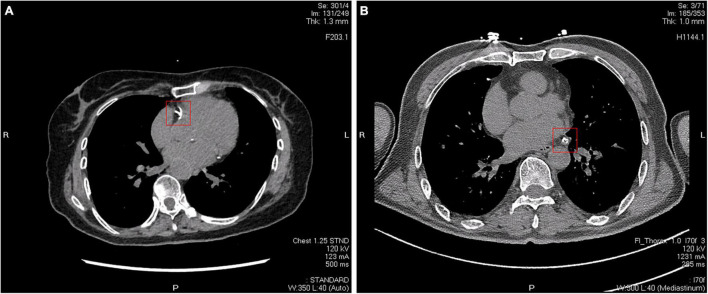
Two different CT images of coronary artery calcification. (A) Calcifications in the right coronary artery indicated by a red square. (B) Calcifications in the circumflex branch of the left coronary artery indicated by a red square.
